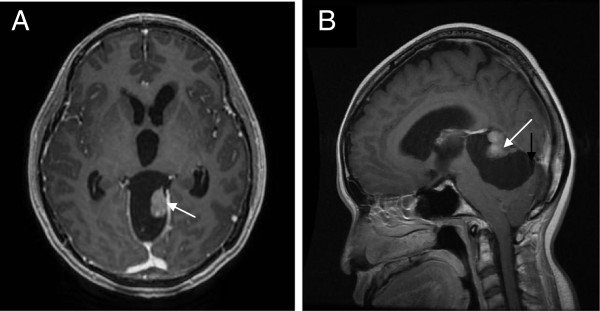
Contrast-enhanced T1 axial (A) and sagittal (B) magnetic resonance imaging demonstrating the extra-axial posterior fossa cystic lesion from the posterior interhemispheric fissure (black arrow) with an enhancing mural nodule (white arrow). No evidence of a dural tail was seen. Also noted were dilated 3rd and lateral ventricles.
